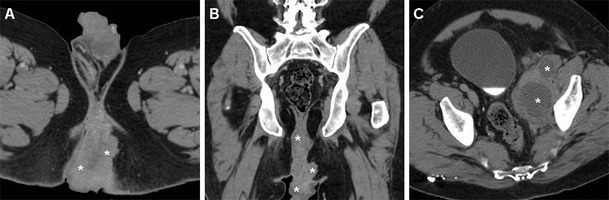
A 60-year-old man with AIDS and clinical finding of ulcero-fungating anal mass. Axial (a) and coronal reformatted (b) CT images show moderately heterogeneous tissue (*) in its entire longitudinal extent from the anorectal junction to below the anal verge, associated with large necrotic iliac adenopathies (* in c). Biopsy confirmed superinfected SCAC
